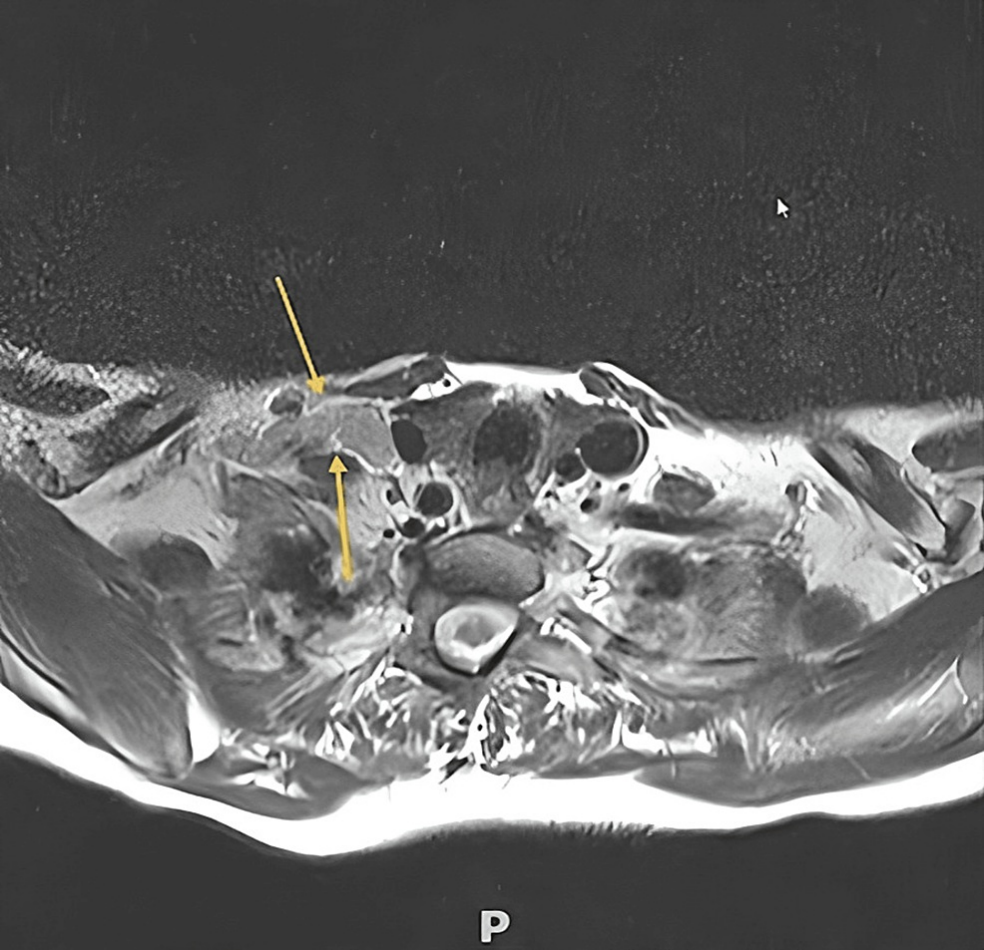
Patient’s neck MRI depicting the pathologically enhanced cervical lymph nodes (yellow arrows) MRI: magnetic resonance imaging
